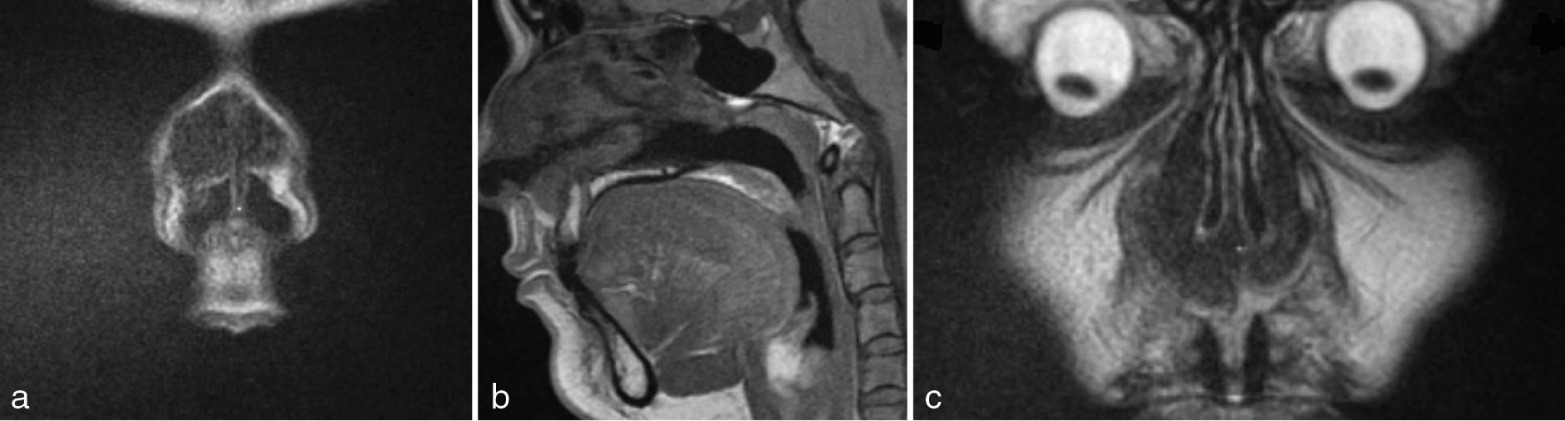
MRI of patient 1. (a) Coronal *T*
